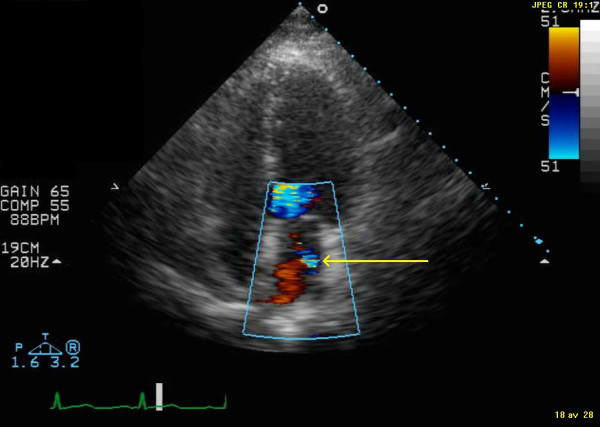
Display of end-systolic image from apical 4-chamber view six months after discontinuation of pioglitazone. The yellow, thinner, arrow indicates the remaining mild mitral regurgitation.
